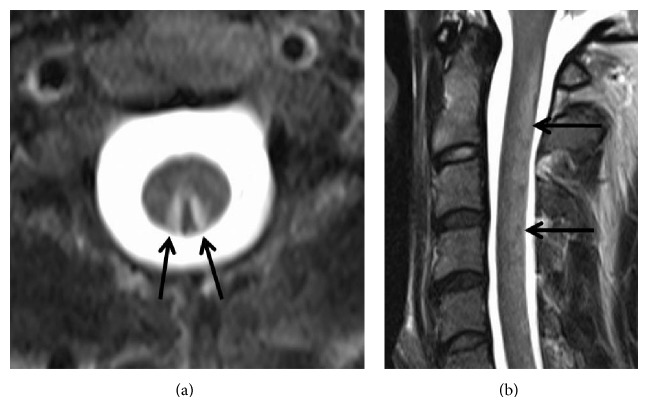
The T2-weighted axial (a) and sagittal (b) images of the cervical spine show hyperintensity (arrows) of the dorsal columns of the spinal cord. There was no contrast enhancement of the lesion (not shown).
